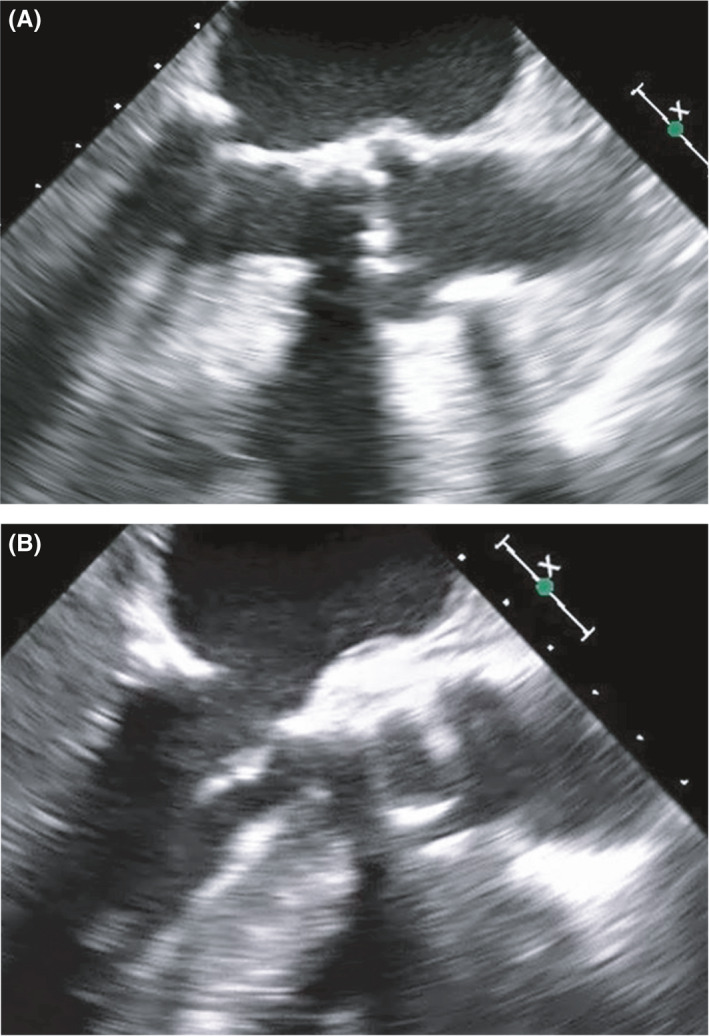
Pre‐ and post‐procedure transesophageal echocardiography in the diastole phase. (A) Pre‐procedure (125 degrees). (B) Post‐procedure (125 degrees). Compared with the pre‐procedure anterior mitral leaflet (A), the post‐procedure anterior mitral leaflet more extensively and smoothly opened in the diastole phase (B)
